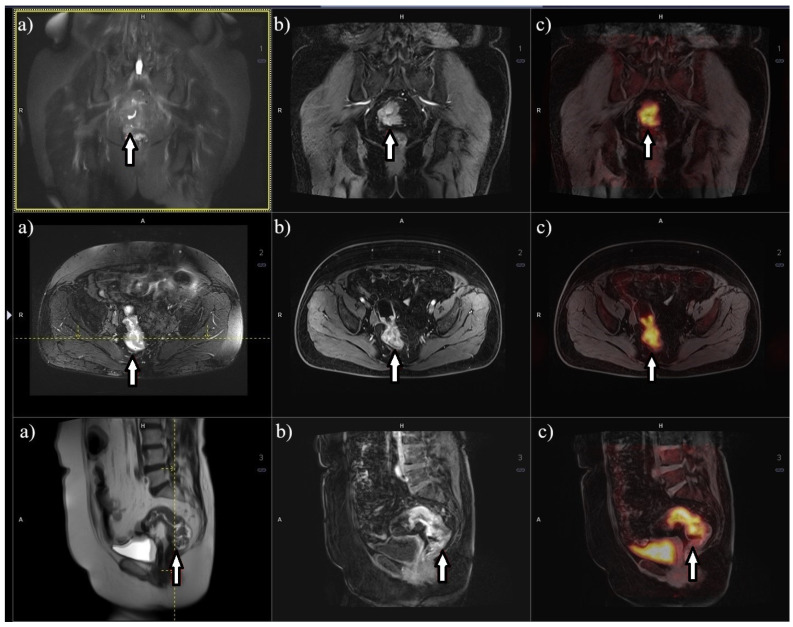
PET/MR images on a 3-tesla Biograph mMR Siemens^®^ scanner using fluorodeoxyglucose labeled with fluorine (18F-FDG). The images show rectal cancer (arrow), respectively: (a) MR in T2-weighted sequence, (b) MR in T1-weighted sequence, and (c) fusion of MR images in T1-weighted sequence and PET.
